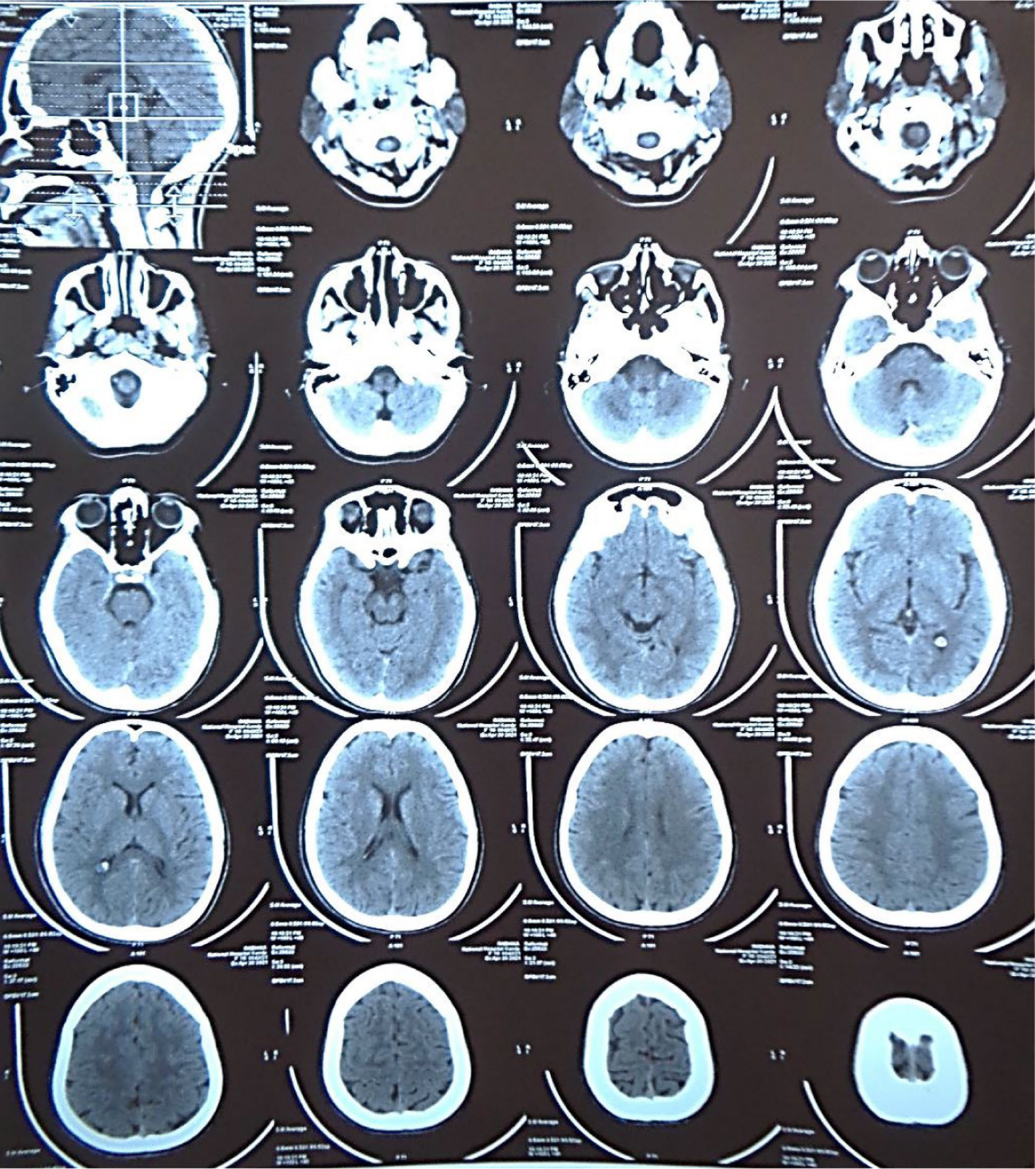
Noncontrast computed tomogram of brain at presentation, reported as normal
